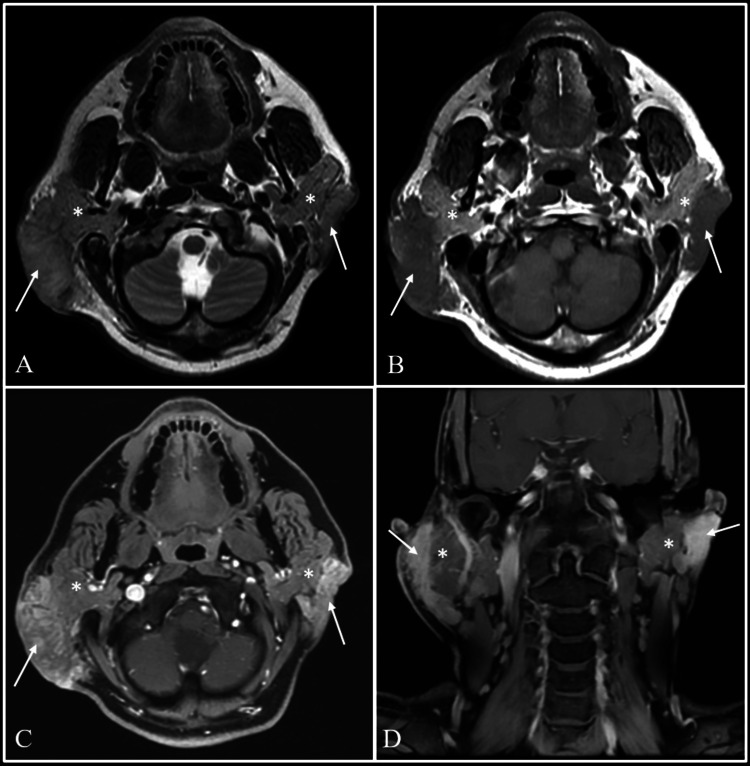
Evaluation with MRI Magnetic resonance imaging (MRI) with axial T2-weighted (A), axial T1-weighted (B), axial and coronal post-gadolinium T1-weighted images (C and D) showing T2 intermediate intensity lesions with heterogeneous post-contrast enhancement in bilateral infraauricular and retroauricular regions (arrows). The lesions are seen superficial to and separately from both the parotid glands, which is best appreciated on Pre-Gadolinium T1-weighted images (B). The parotid glands show normal signal intensity on both sides (asterisks).
